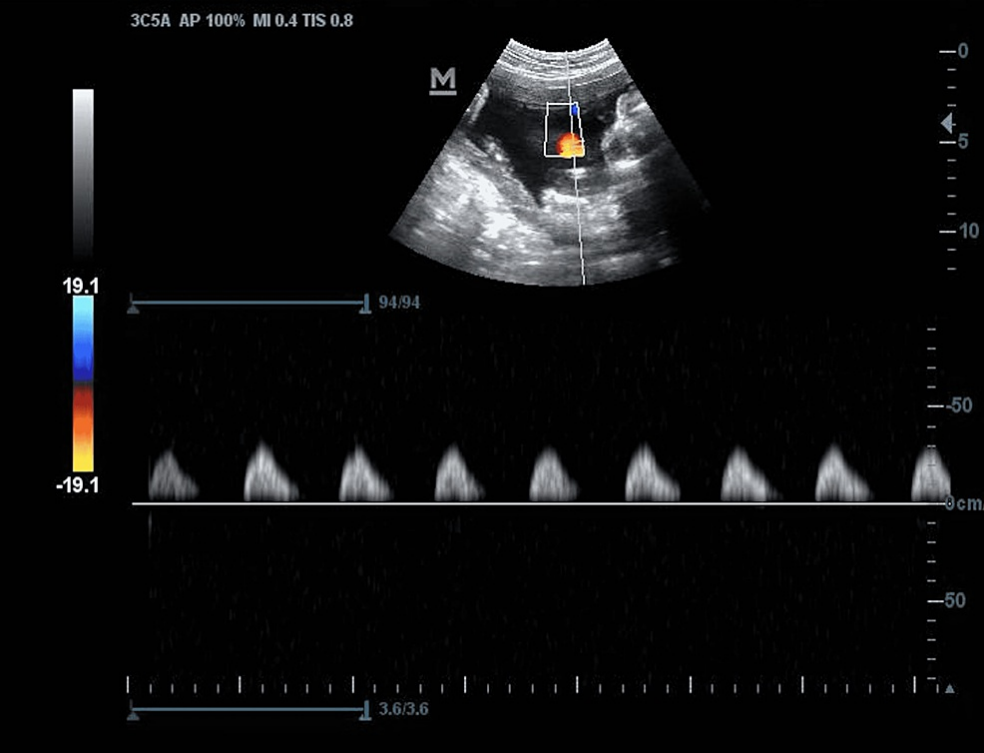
Absent end-diastolic flow in the umbilical artery on Doppler ultrasonography
